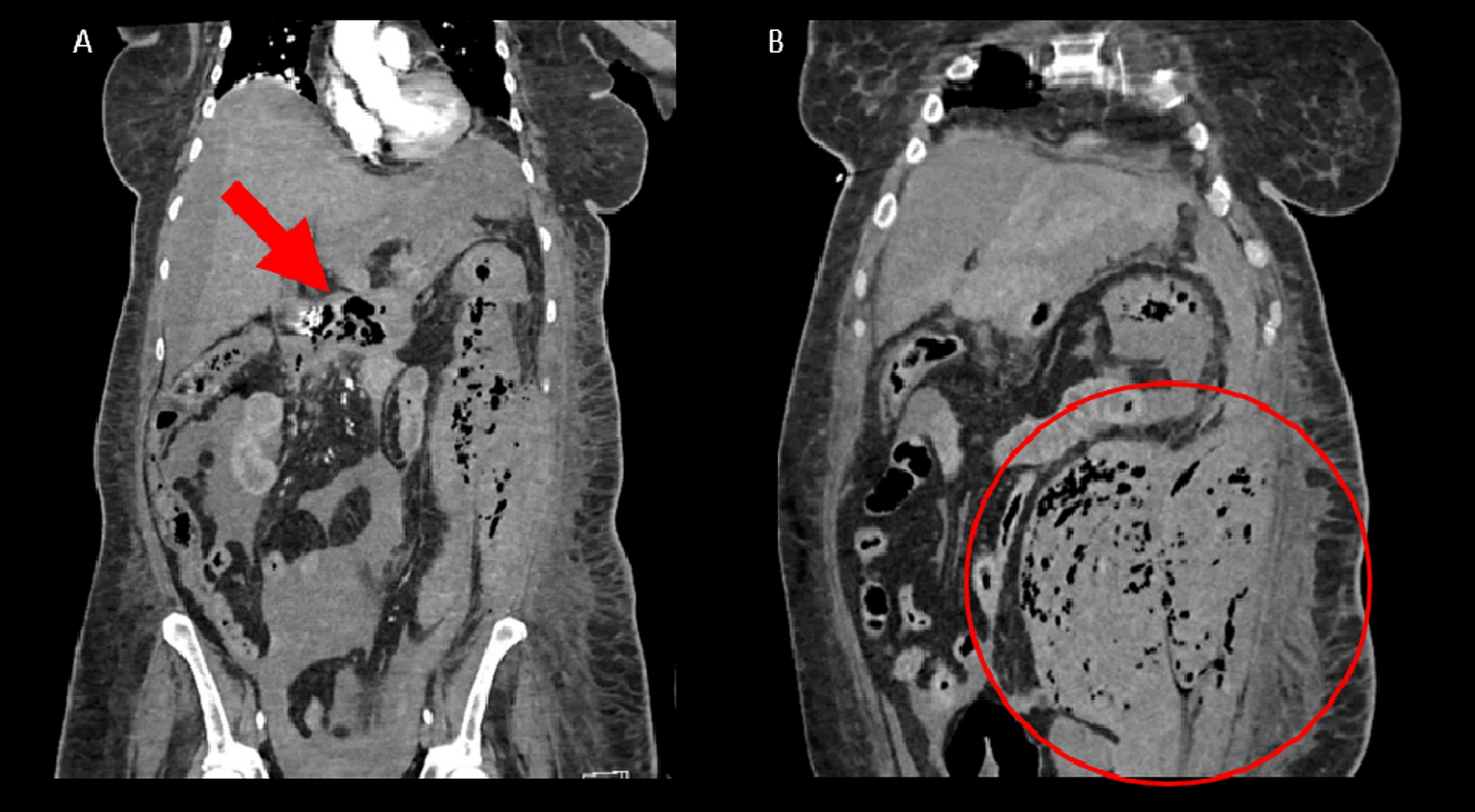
Coronal views of CT angiography revealing (a) emphysematous pancreatitis with (b) hemoperitoneum. CT, computed tomography
